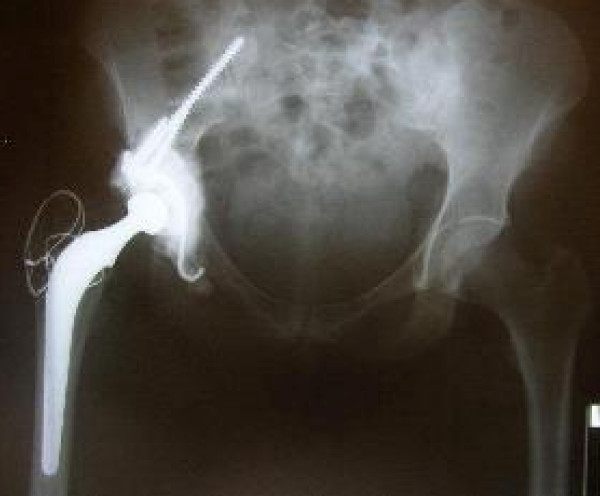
Radiograph after Right total hip replacement.
